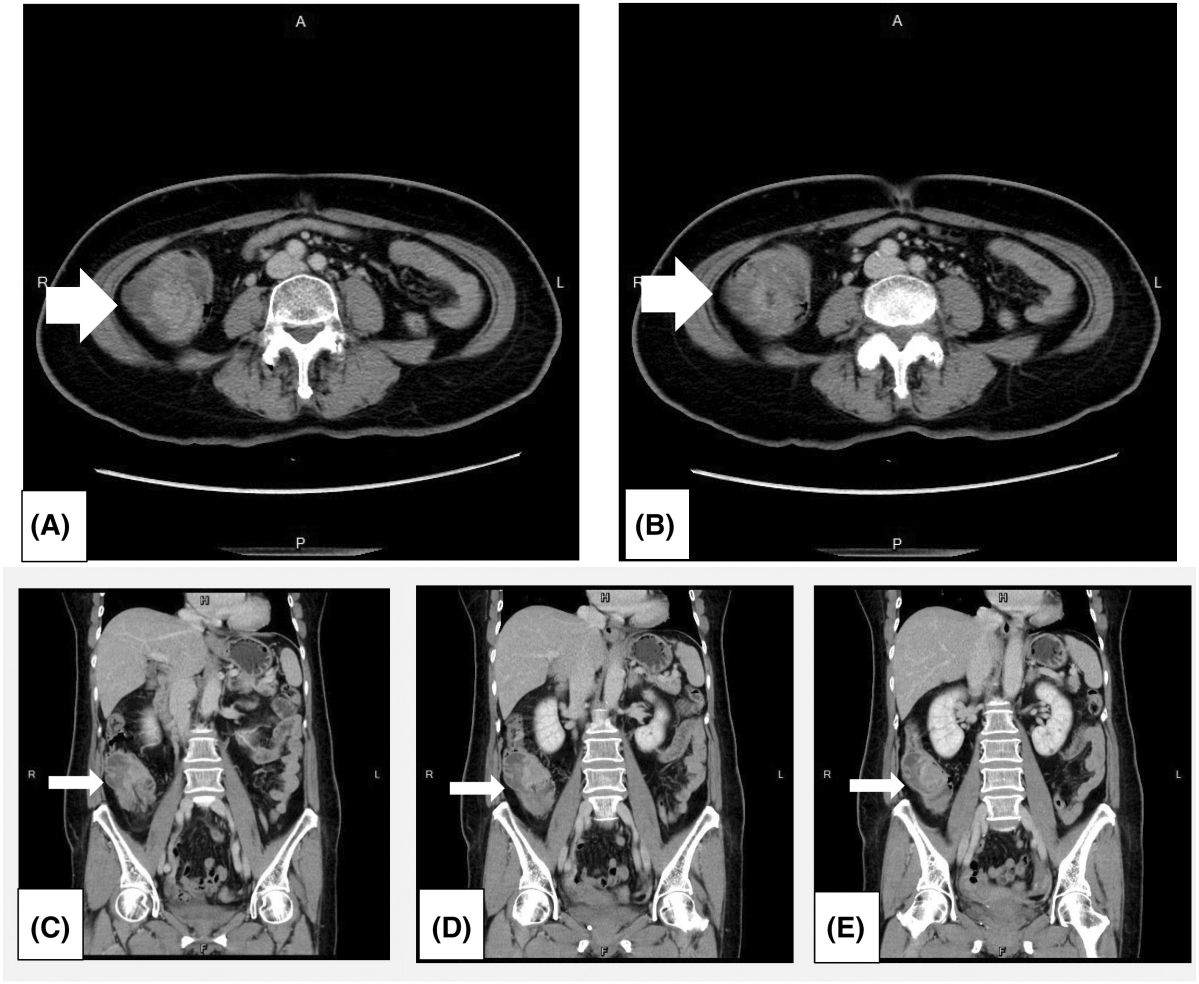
Abdominal Computed Tomography (CT) scans provided multiple perspectives of the intussusception: (A) Axial View: The axial view displayed the classic ‘target sign’, which is characteristic of ileocolic intussusception. (B) Reniform Mass Lesion: A reniform mass lesion was observed, attributed to edematous changes and increased mural thickness. (C, D, & E) Coronal Views: Coronal views provided additional confirmation of ileocecal intussusception, as indicated by the directional arrows.
